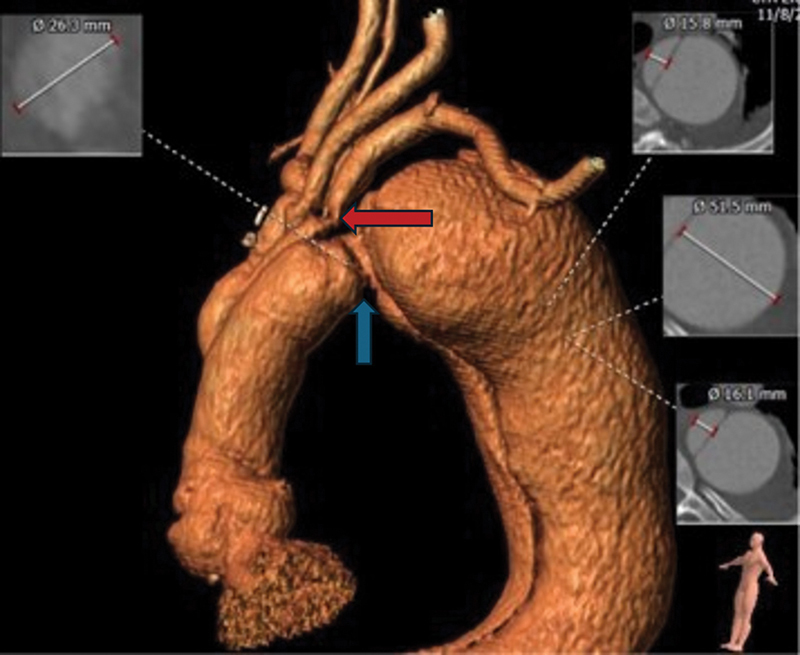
Computed tomographic angiogram after repair of Type A dissection and prior to repair of Type B aortic dissection. There is a stenosis at the graft to native aortic anastomosis (blue arrow) and a stenosis at the junction of the graft to left subclavian artery anastomosis (red arrow).
